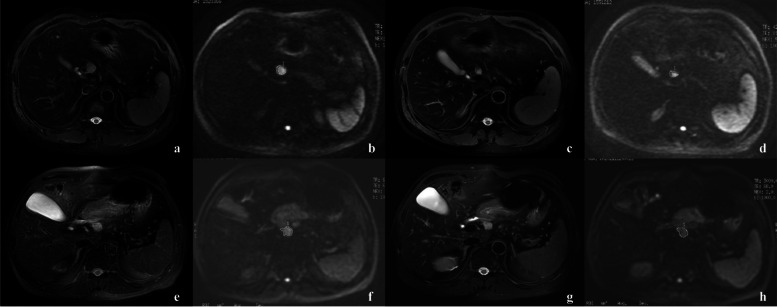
Two examples of MRI images from CRLM patients, including the T2WI and DWI before and after treatment. a-d A short diameter of HLN shrinkage from 16 mm (a) to 11 mm (c). The ADC value calculated from the DWI sequence (b, d) slightly increased from 1140 to 1230 mm^2^/s. The lymph node was pathologically confirmed to be a metastatic lymph node. e-h A short diameter of HLN shrinkage from 15 mm (e) to 12 mm (g) after treatment. The ADC value calculated from the DWI sequence (f, h) increased from 1330 to 1650 mm^2^/s. The lymph node was pathologically confirmed to be a non-metastatic lymph node
